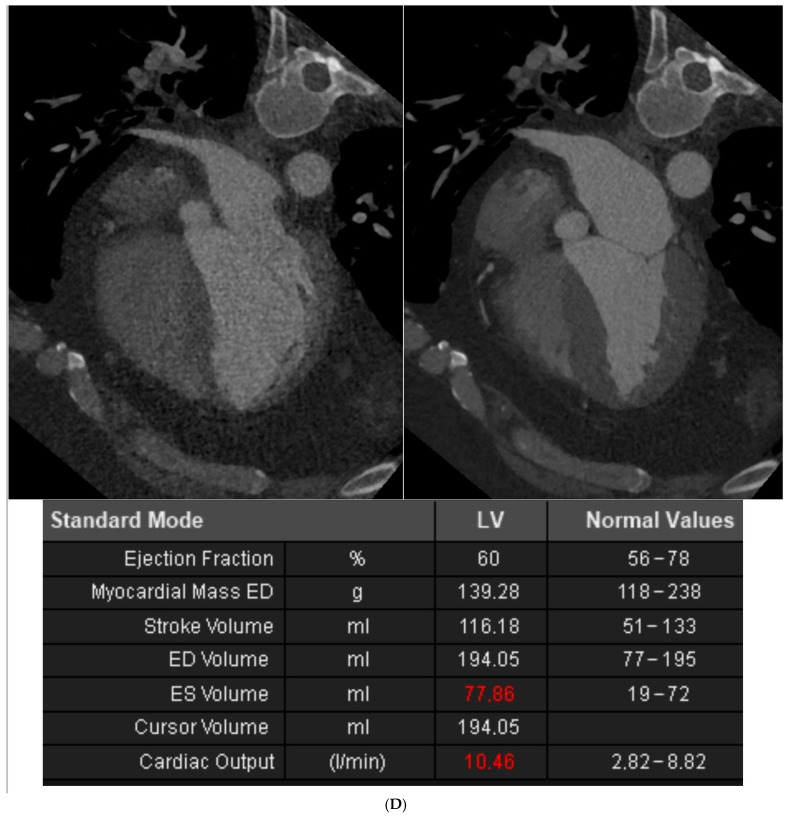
Case 2. A high CACS without significant coronary artery stenosis in CCTA. A 57-year-old male patient was referred for computed tomography angiography of the coronary arteries from the outpatient cardiology clinic due to numerous risk factors for cardiovascular disease in their medical history and sudden cardiac death in the family. CCTA examination was performed according to the standard protocol. (A) The native phase of CCTA revealed the presence of calcified changes in the topography of coronary arteries. The calcium index of individual coronary arteries calculated using the Agatston method was LM 0, LAD 403, LCx 207.7, RCA 12. The CACS was 622.7, representing a significant population risk of significant CAD. (B) Computed tomography volumetric reconstruction technique. The quality of the obtained images of the angiographic phase of the CCTA examination was assessed as average. The average quality of CCTA images was the result of the patient’s obesity and fast heart rate during acquisition.). (C) Curved multiplanar reconstructions (cMPR). LM was short, without stenosis. In the proximal and middle sections of the LAD, numerous calcified and mixed atherosclerotic plaques with positive remodeling were visible, causing stenosis of 25–50%. Similarly, in the proximal and middle sections of the LCx, numerous calcified and mixed atherosclerotic plaques with positive remodeling were visualized, causing stenoses of 25–50%. Calcified atherosclerotic plaques were found in the RCA, causing stenosis <25%. The entire CCTA image met CAD-RADS 2 criteria. (D) Assessment of left ventricular functional parameters. The left ventricular ejection fraction was 60%, the left ventricular end-diastolic volume was 194.05 mL, and the left ventricular mass was 139.28 g. The left ventricle was not enlarged and had normal global systolic function. In further diagnostic and therapeutic procedures, due to the occurrence of increasing chest pain, it was decided to verify the coronary arteries via invasive coronary angiography. Invasive coronary angiography excluded significant stenoses in the coronary arteries.
